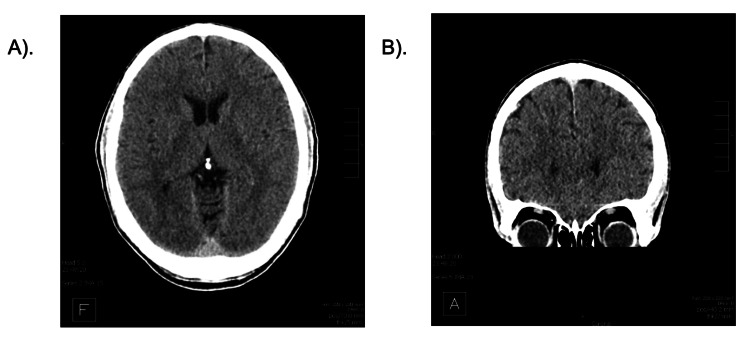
Computed tomography of the head without contrast in transverse plane (A) and coronal plane (B).
